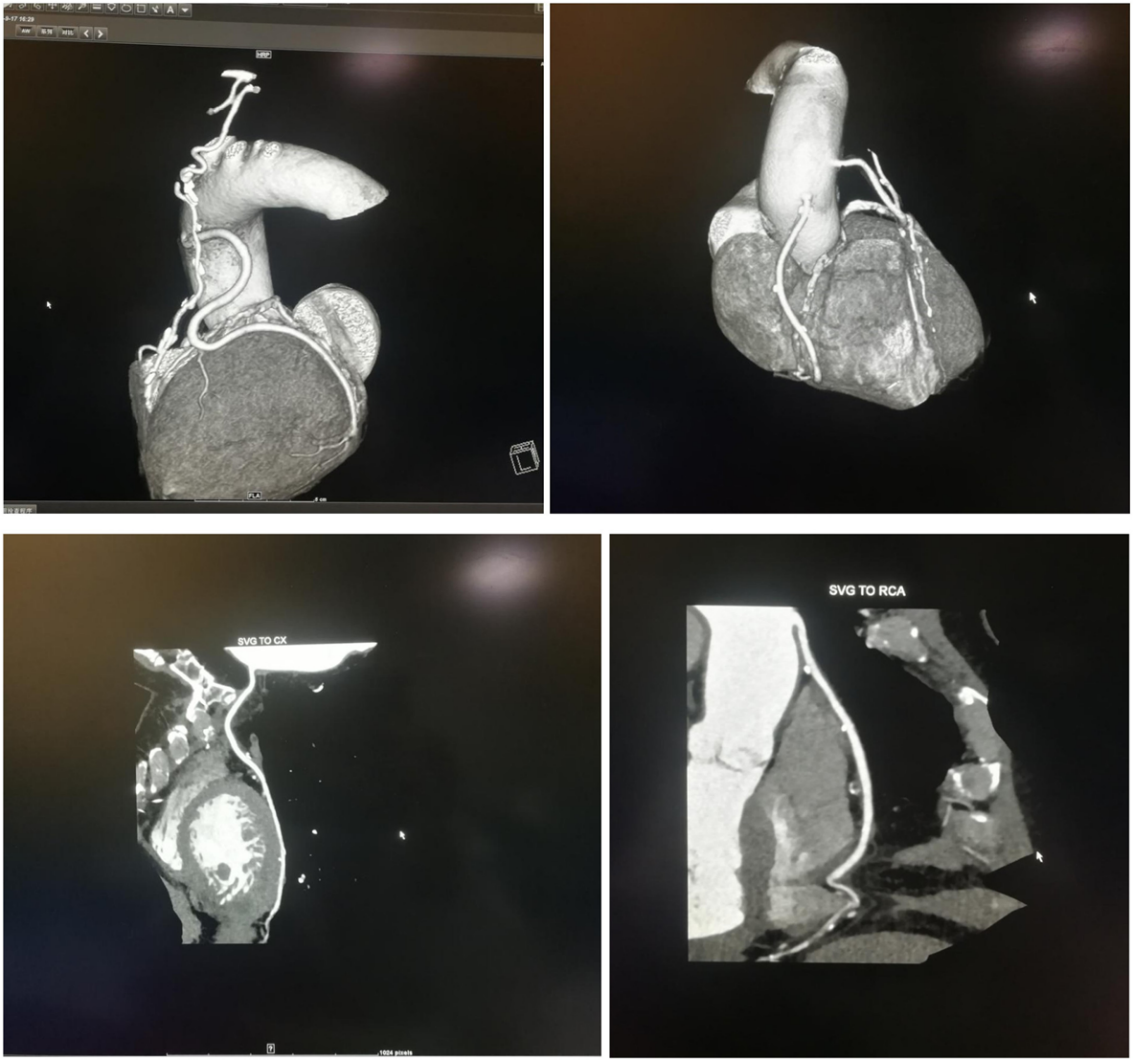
Vascular CTA after CABG in MVH
